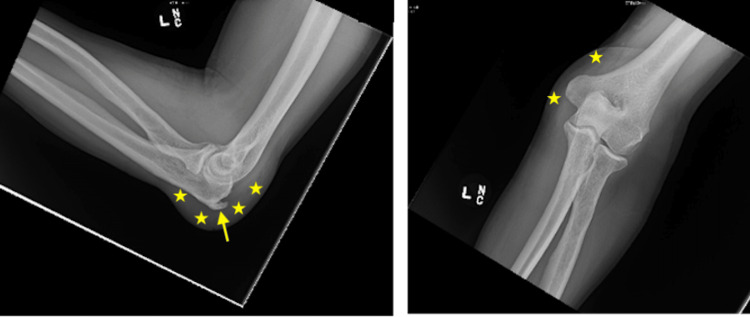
Plain radiographs of the left elbow demonstrating an olecranon spur (arrow) and inflammation of the fluid-filled bursa (stars).
